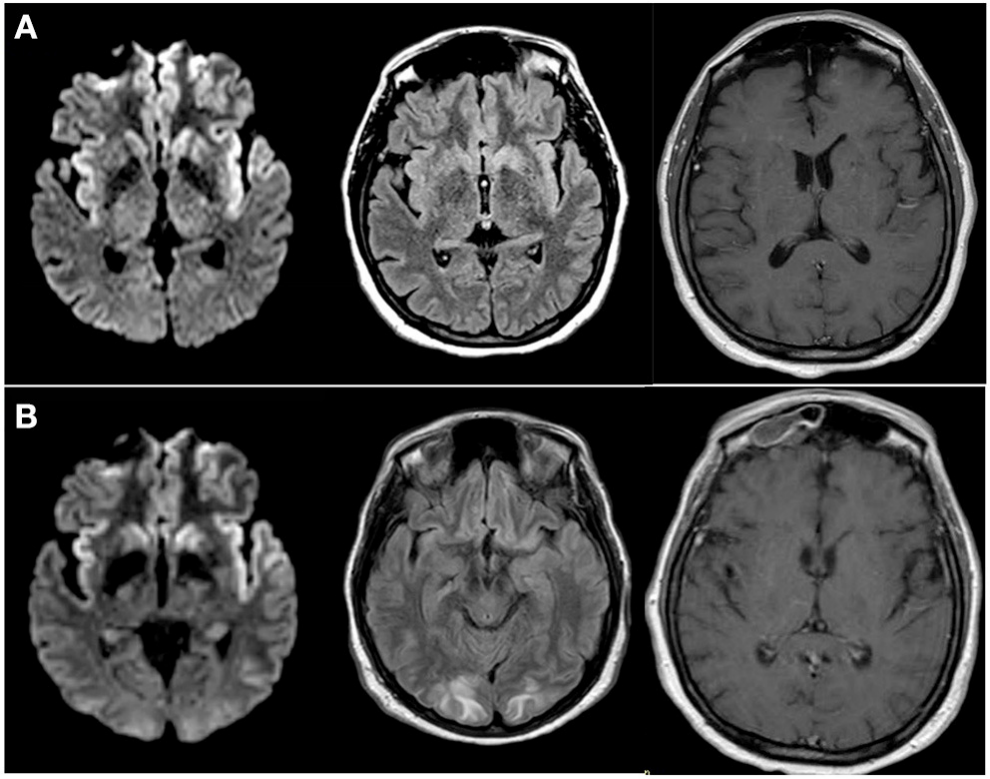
Progression of MRI Findings (A) MRI brain with and without intravenous contrast; Axial diffusion-weighted imaging (DWI) (left), FLAIR (middle) and T1 post-contrast (right) images obtained at the time of initial presentation to our institution demonstrated cortically-based increased signal within the paramedical frontal lobes and insular cortices bilaterally. Additional diffusion restriction is present within bilateral caudate heads and the left putamen. (B) Follow up MRI brain without contrast; axial DWI) (left), FLAIR (middle) and T1 post-contrast (right) images obtained 4 days later demonstrate unchanged cortically-based and basal ganglia diffusion restriction. FLAIR images also reveal new patchy subcortical white matter hyperintensity with involvement of the subcortical U-fibers. Contrast enhancement of pachymeninges seen diffusely.
